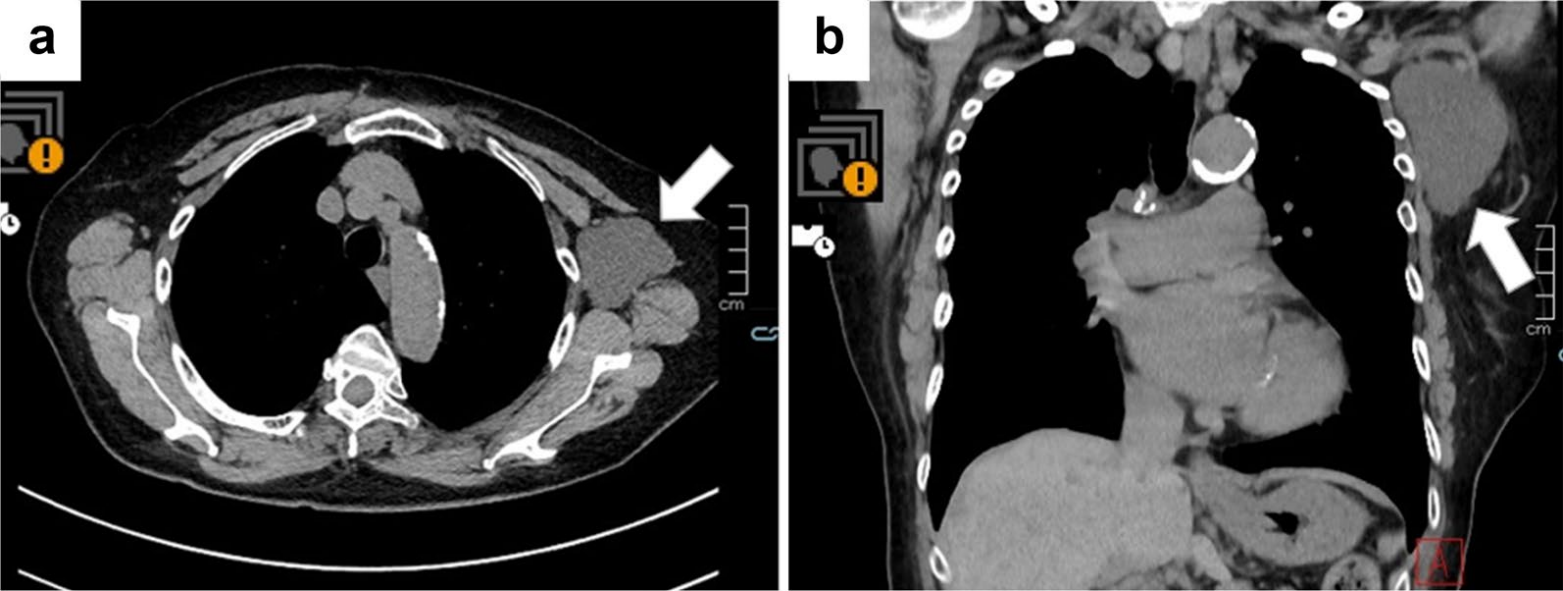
Non-contrast-enhanced computed tomography (NECT) findings (axial and coronal). Axial (a) and coronal (b) NECT scans showed a homogeneous, low-attenuation mass in the left axilla. The mass is slightly lobulated
